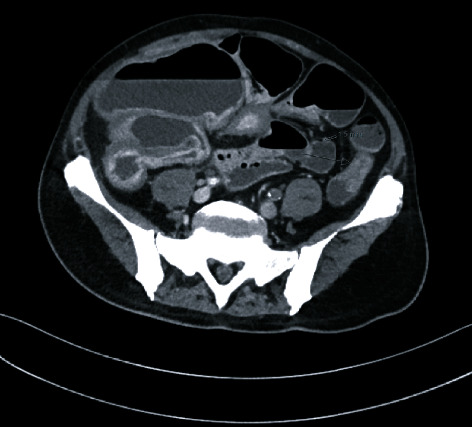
Abdominal CT scan taken at the L5 level, demonstrating focal mucosal thickening and dilation of the ascending and proximal descending colon.
